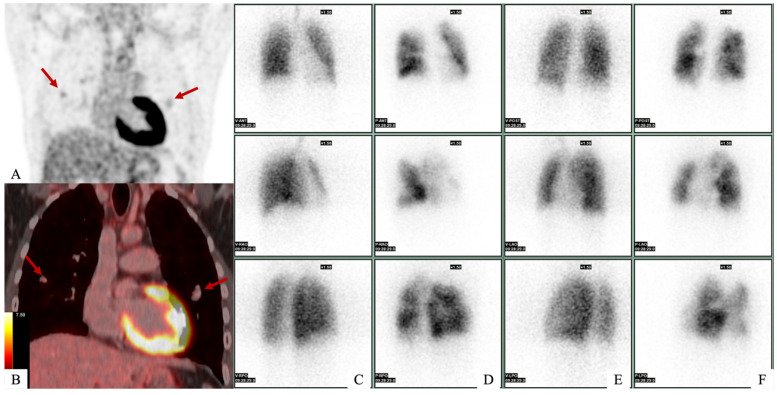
A 21-year-old man with no medical history presented to the emergency department with dyspnea and asthenia. Initial investigations included transthoracic echocardiography, which revealed a mass in the right atrium, and right heart catheterization, which confirmed pulmonary hypertension. Computed tomography (CT) confirmed the presence of a mass in the right atrium in contact with the tricuspid valve, as well as multiple bilateral pulmonary emboli and aneurysms. A complete work-up was carried out. (A,B) FDG-PET scan revealed mild intra-pulmonary hypermetabolism, which corresponded with pulmonary arterial aneurysms (*red arrows*), for which the SUVmax was measured at 2.4 with an aortic background noise of 2.6. (C–F) Ventilation/perfusion pulmonary scintigraphy revealed several bilateral perfusion defects on anterior (D) and posterior (F) views with systemized areas and other areas of hyperperfusion. There were no ventilation defects in both lungs on anterior (C) and posterior (E) view.
